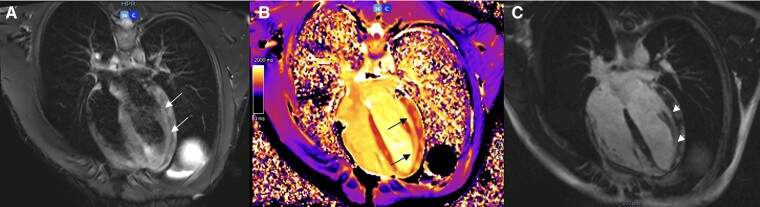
Cardiac magnetic resonance imaging images (four-chamber view) of Patient 1, consistent with acute myocarditis (Lake Louise Criteria fulfilled). (*A*) Short tau inversion recovery image shows several areas of hyperintensity on the lateral wall of left ventricle (white arrows), indicating myocardial oedema. (*B*) T1 mapping image depicts multiple regions of increased myocardial T1 relaxation times (black arrows), reflecting myocardial injury. (*C*) Late gadolinium enhancement sequence shows extensive non-ischaemic late gadolinium enhancement on the same locations (arrowheads), indicating myocardial fibrosis.
